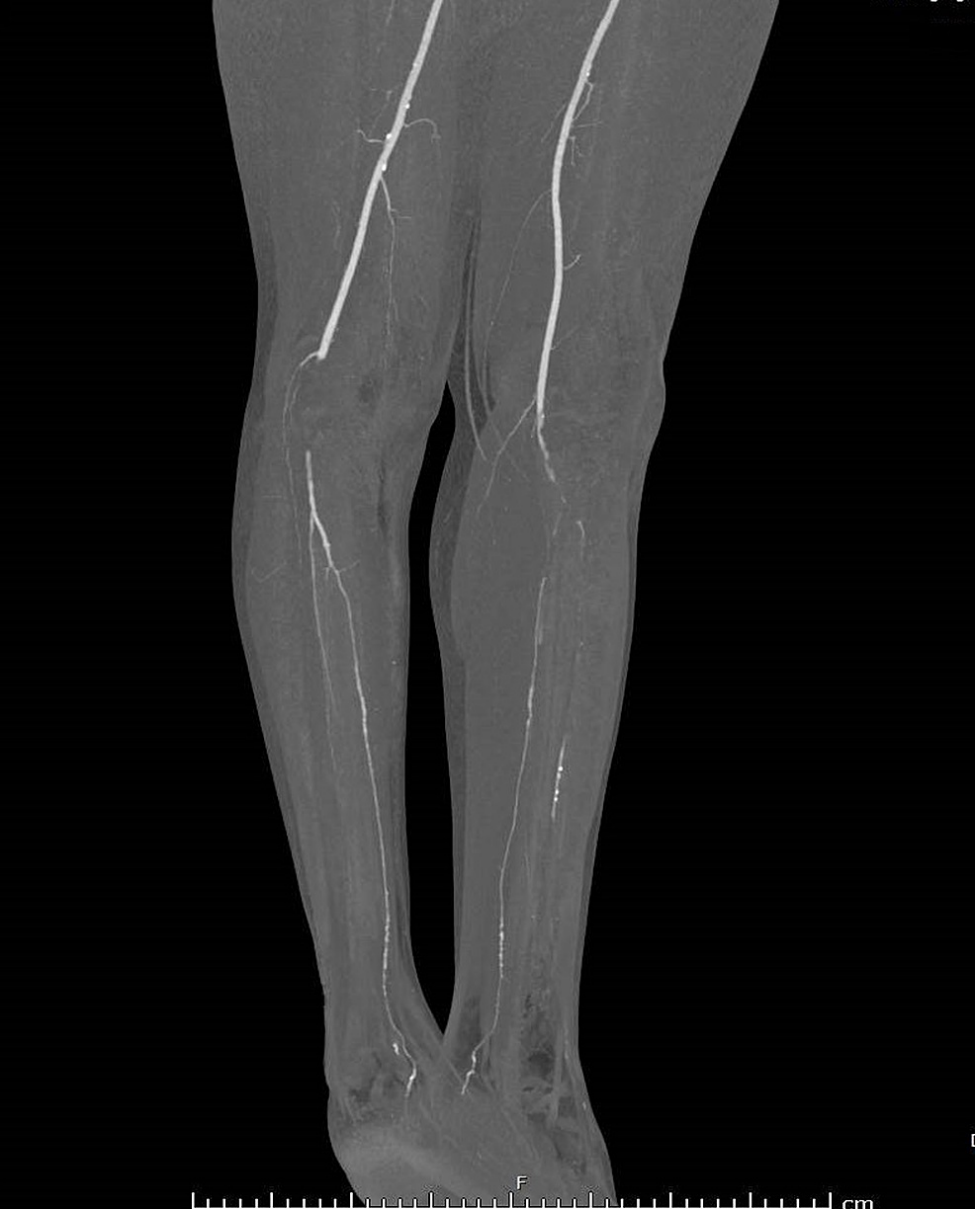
CT angiogram showing right and left-sided popliteal artery occlusion (lateral view).
